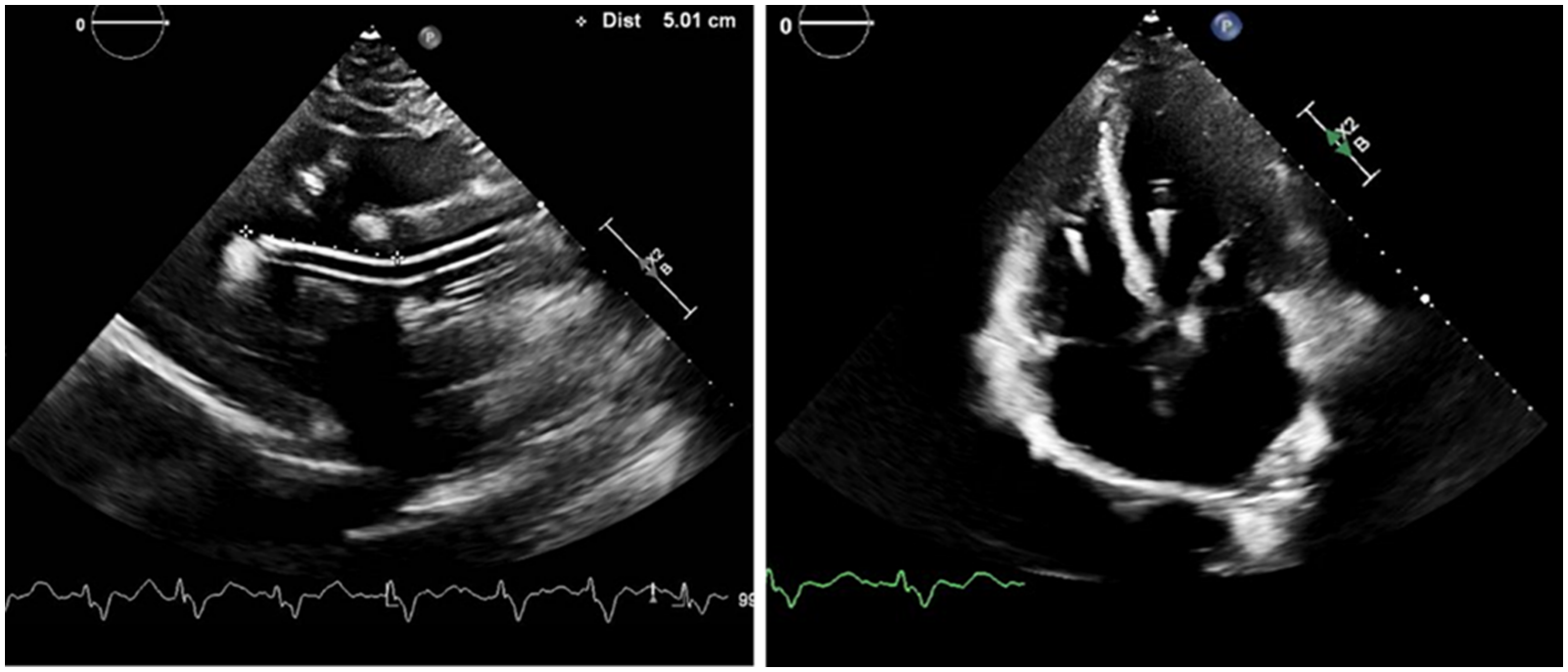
Positioning of the Impella 5.5: The bending portion of Impella pump head will be on the level of aortic valve with the tip pointing away from the posterior wall in the parasternal long axis view, and away from septum in the four chamber view. Impella position-related aortic insufficiency could be observed for patients with atherosclerotic aortic valve in the apical view.
